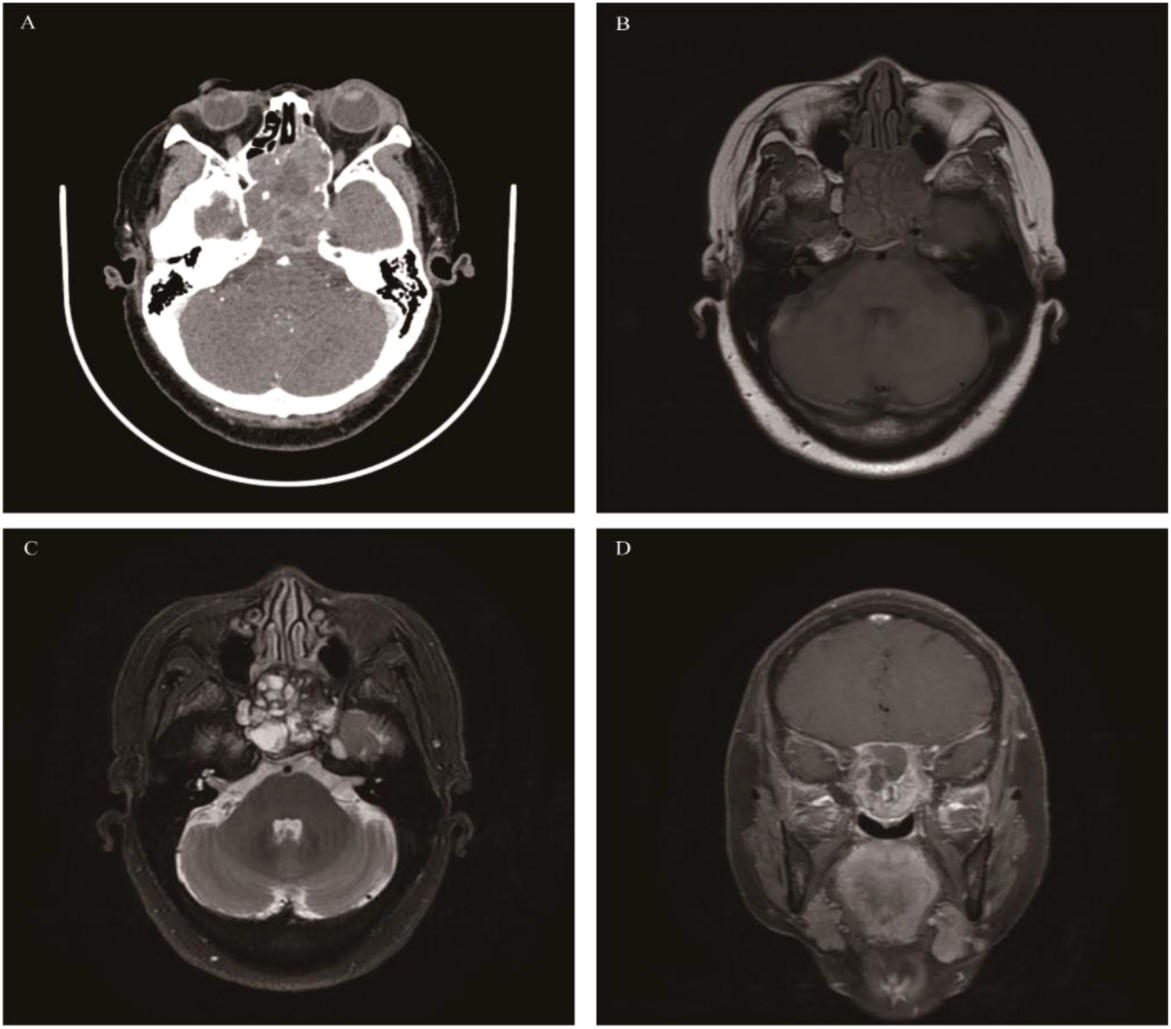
Giant cell tumor of bone: Axial CT enhancement revealed an irregular, hyperintense mass causing extensive bone damage in the pterygoid sinus, slope, and posterior sieve sinus (A). The mass displayed the typical “soap bubble-like” appearance on T1WI and T2WI, akin to GCTB found in the metaphysis of long bones (B, C), with the solid part of the mass demonstrating significant enhancement on coronal fat-saturated contrast-enhanced T1WI, while the liquid component does not (D).
